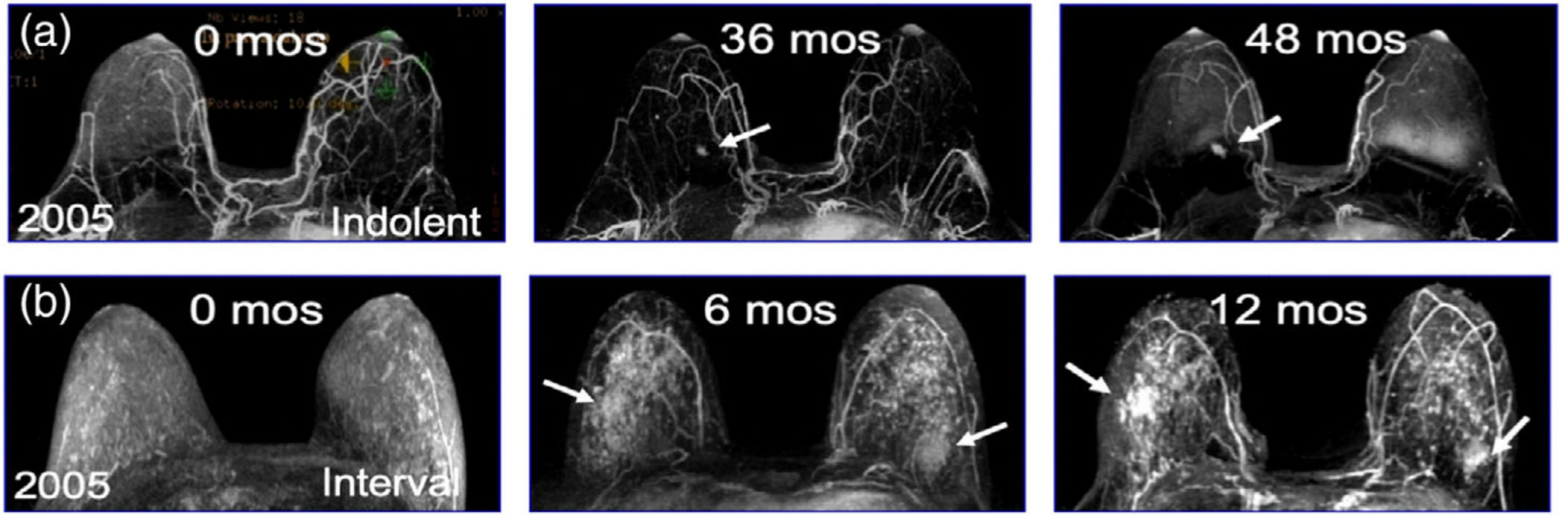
Screening breast MRI imaging of slowly progressing (a) versus rapidly progressing interval (b) breast cancer. (a) Slowly progressing ER/PR+ HER2-wild-type breast cancer; arrow at 36 months indicates when breast cancer was first observed, however at that time, it was too small for our clinical team to biopsy; arrow at 48 months demonstrates slow growth of the tumor over the 12 month interval; the breast cancer was biopsied at that point, staging revealed a T1N0M0 indolent breast cancer (b) Rapidly progressing ER/PR– HER2-wildtype triple negative breast cancer (TNBC), 0 months is a normal MRI, at 6 months a change in MRI patterning was observed, this was one of our first bilateral screening MRI and our team did not recognize the importance of this change and recommended a 6 month follow-up, biopsy at 12 months (see arrows) demonstrated a T2N0M0 TNBC, the woman underwent bilateral mastectomy and chemotherapy, she is currently alive and disease free
